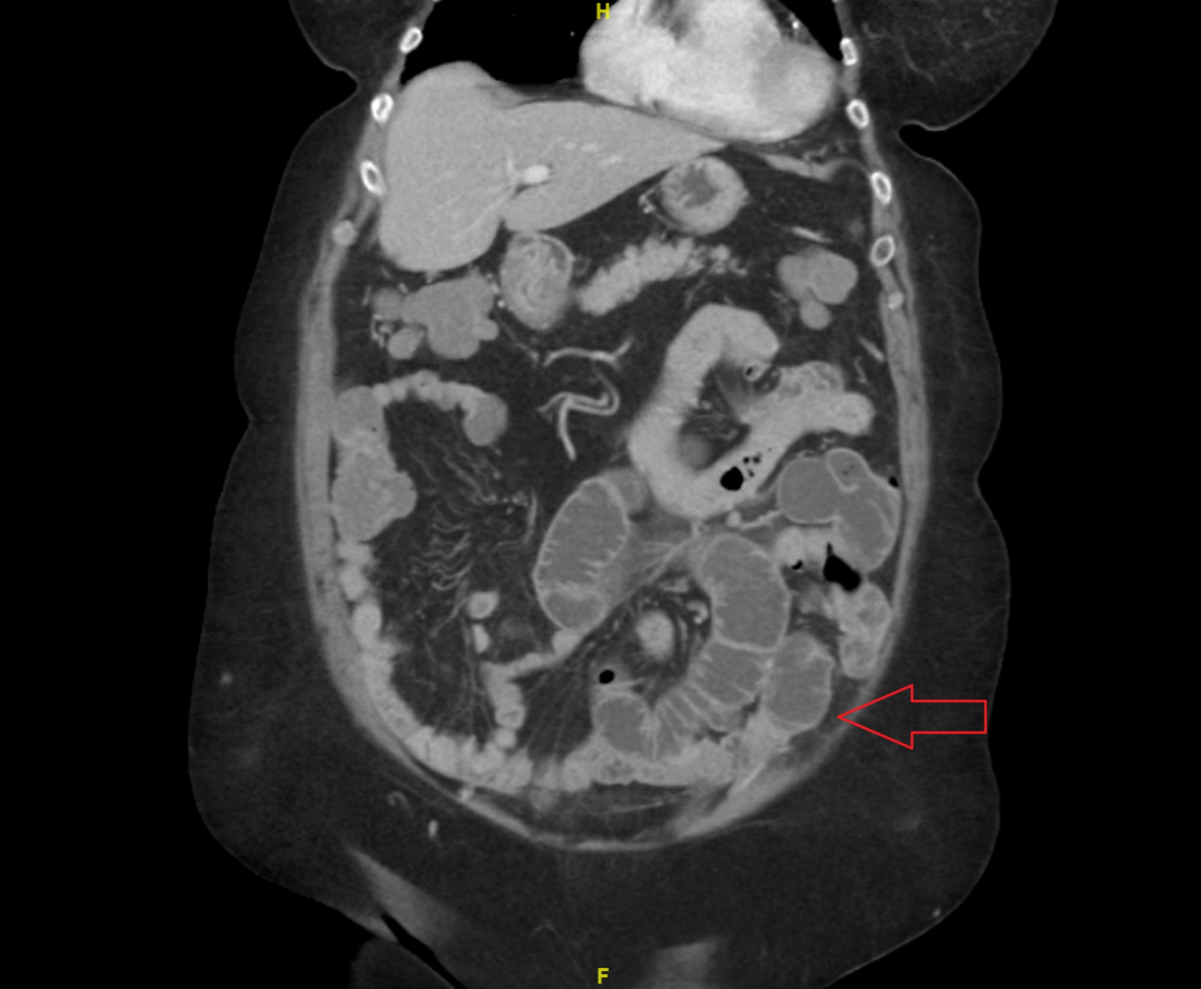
Dilatation of multiple distal loops of the small bowel in the left lower quadrant of the abdomen (abdominal CT with contrast – coronal view)
